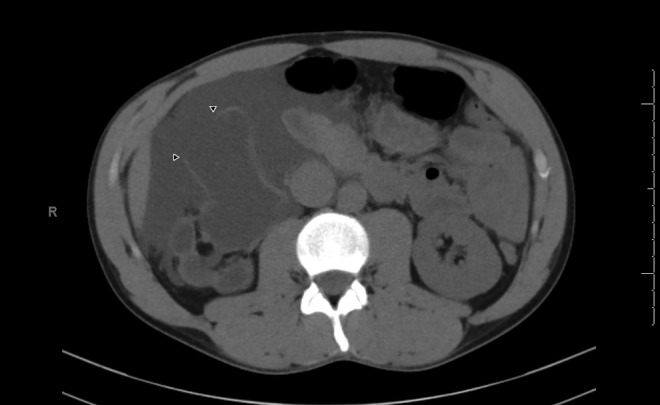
CT abdomen without contrast; axial image of right kidney demonstrating rupture (*arrow heads*) of a severely hydronephrotic pelvis with associated severe cortical atrophy and urine extravasation.
